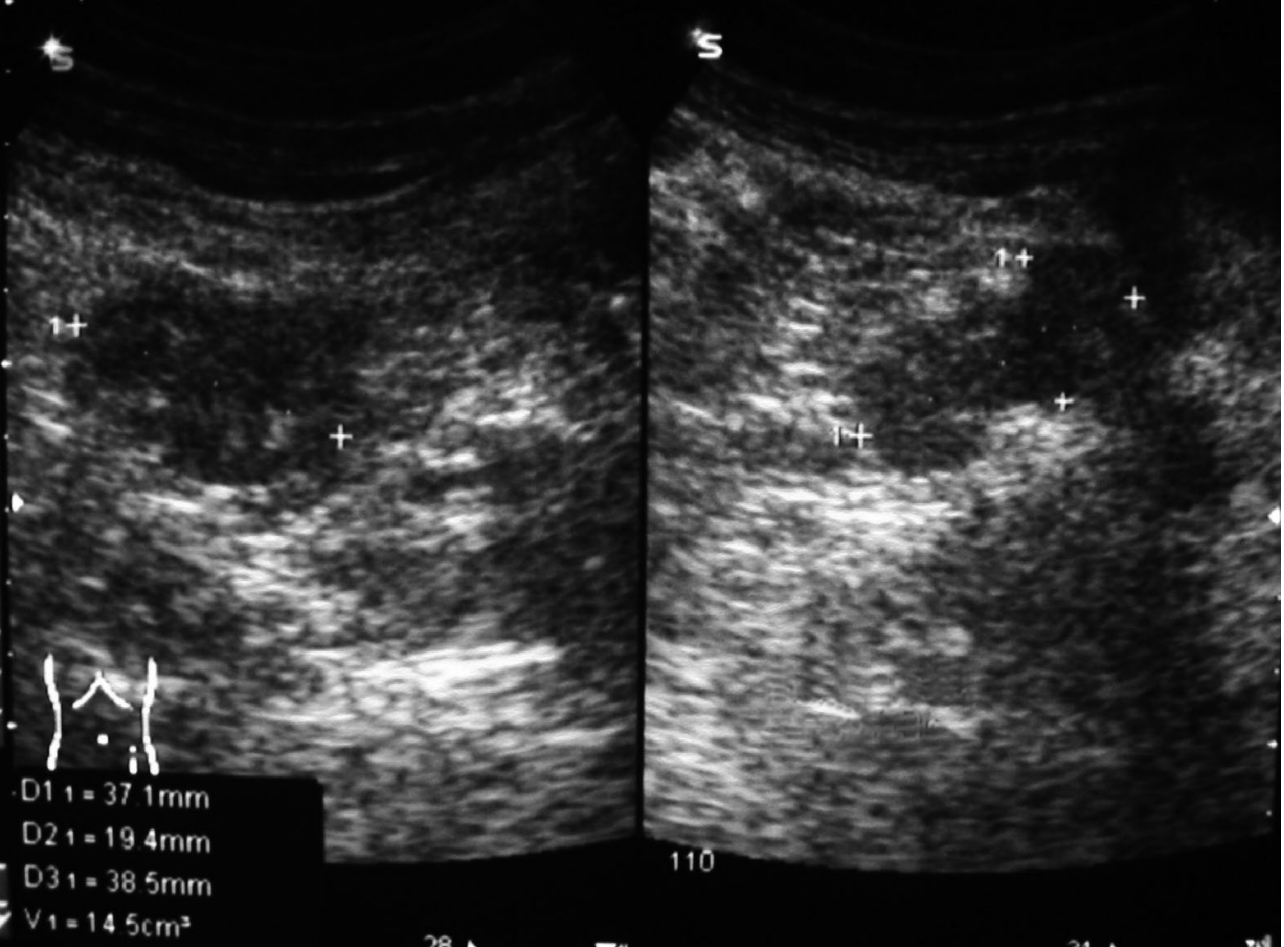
Ultrasound (US) reveals a cystic mass with solid areas in the second case.
